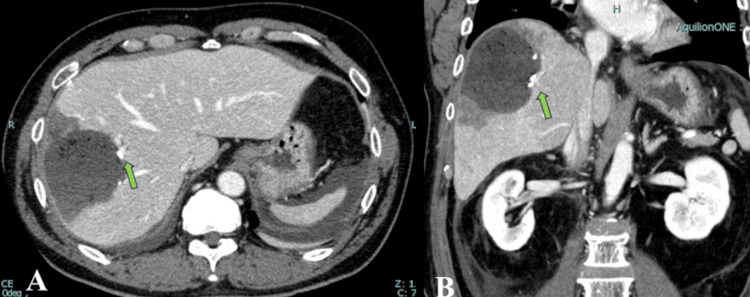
Postoperative images A and B postoperative contrast-enhanced computed tomography findings (green arrows indicate the root of G8 branches). In accordance with the surgical plan, we confirmed that we had successfully resected the root of the G8 branches.
